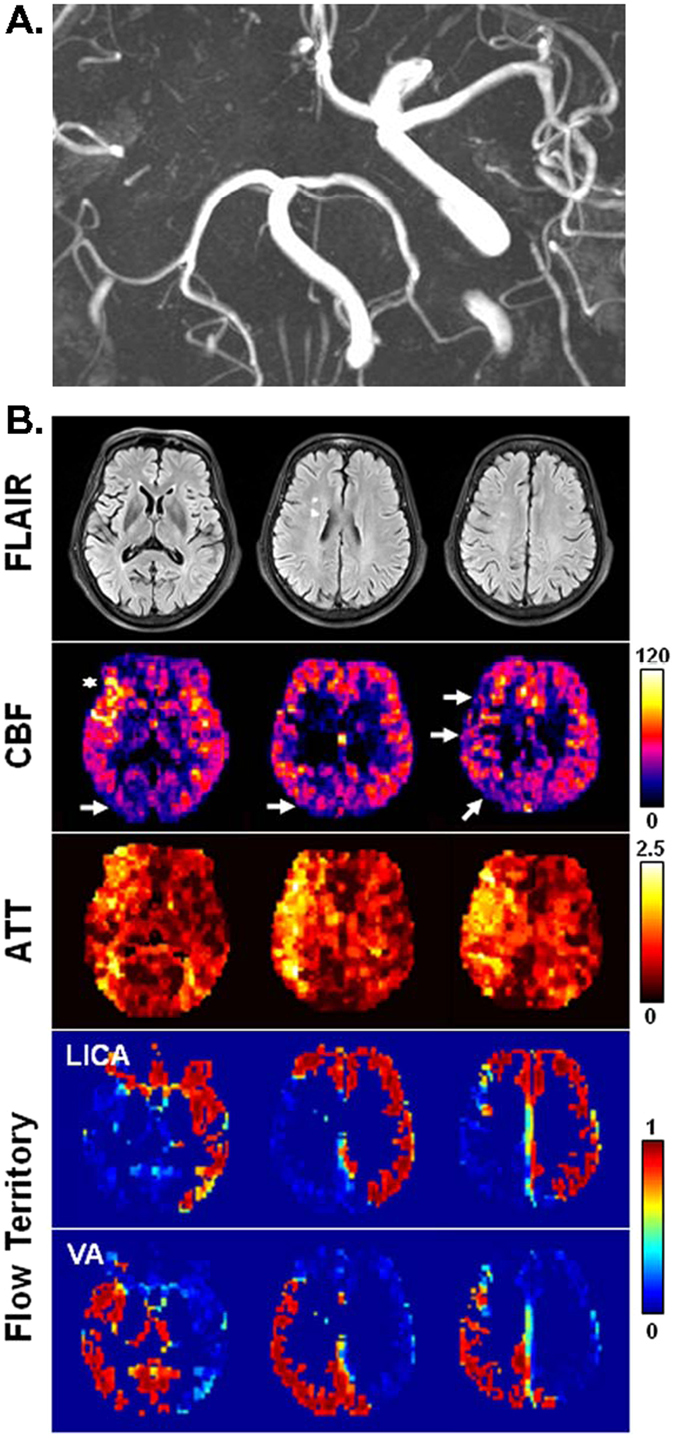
MR images obtained from a 60-year-old female patient with asymptomatic ICA steno-occlusive disease (occlusion in the right ICA and 30–50% stenosis in the left ICA). (A) Time-of-flight angiography. Projection view from below. (B) From top to bottom rows (3 slices are shown in radiological convention): FLAIR images, CBF maps (in units of ml-blood/100 ml-tissue/min), ATT maps (in units of sec), and flow territory maps. Flow territory maps show the membership levels generated by fuzzy clustering, which range from 0 to 1. No contribution from right ICA was extractable. In FLAIR images, there are two small foci of white matter hyperintensity in right frontal white matter which might represent chronic ischemic white matter lesions.
